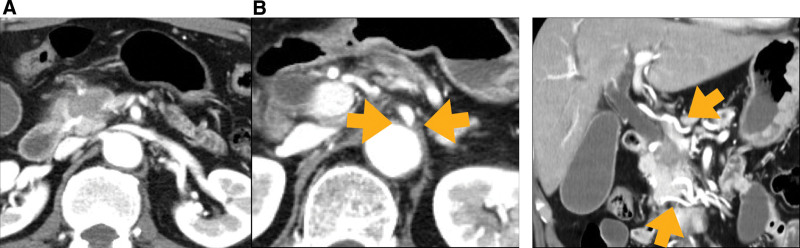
Contrast-enhanced CT scan showing (a) a hypovolemic mass in the pancreatic head and (b) stenosis of the origin of the celiac artery and development of a branch from the SMA (arrows). CT = computed tomography, SMA = superior mesenteric artery.
